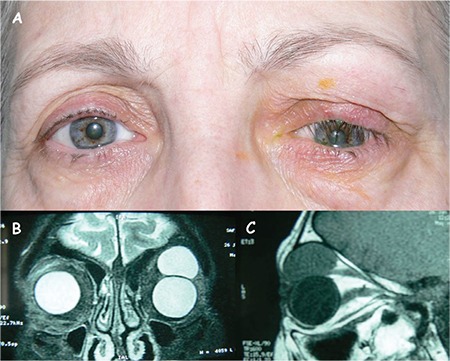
External appearance of the swollen left upper eyelid causing mechanical ptosis (A). On magnetic resonance imaging, an intraorbital, extraconal lesion is evident in both coronal T2-weighted image (B) and sagittal T1-weighted image (C)
